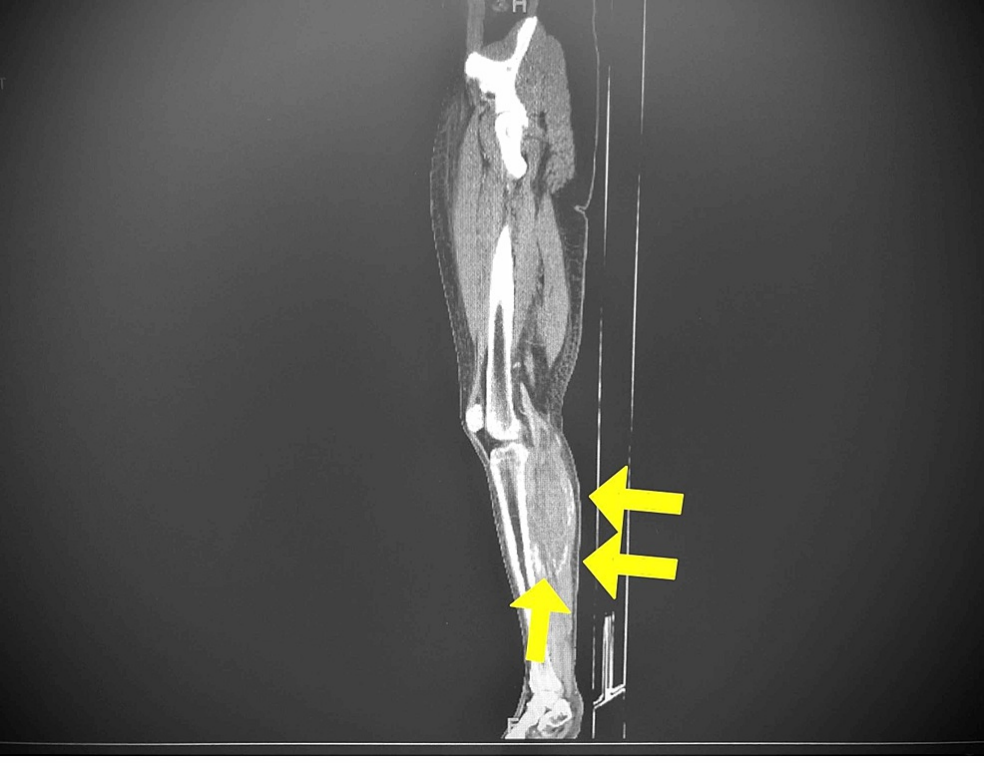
CT (sagittal view) of the left lower extremity. Curvilinear calcifications within the proximal lateral gastrocnemius and soleus muscles.
